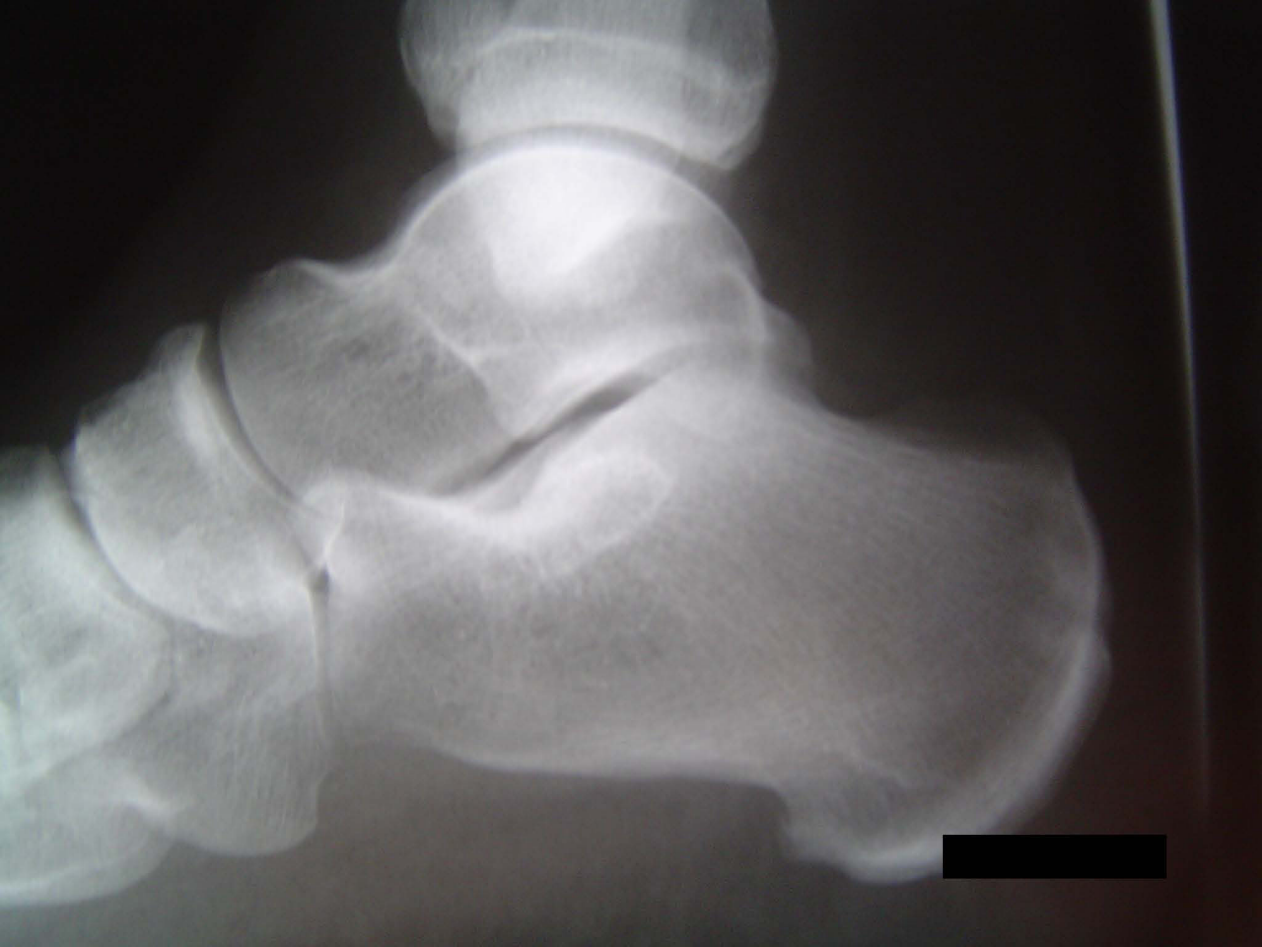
Normal plain radiograph at presentation.
